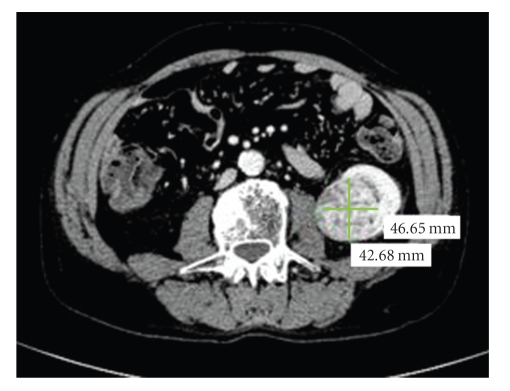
CT scan showing left renal tumor before RIC HSCT.
